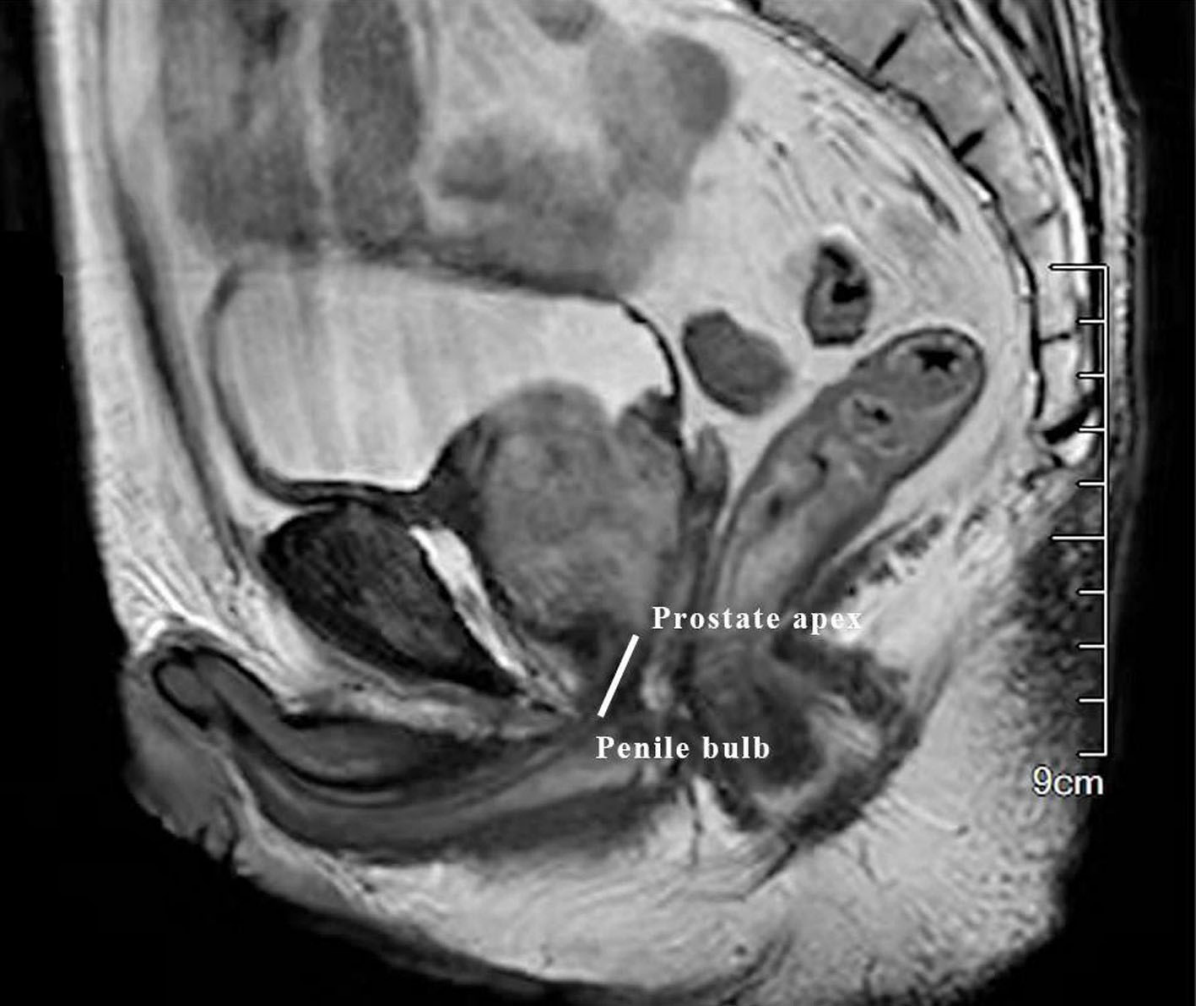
The measurement of membranous urethral length (MUL). The membranous urethral length (MUL) was measured from the prostate apex to penile bulb on the preoperative sagittal plane of T2-weighted MRI.
